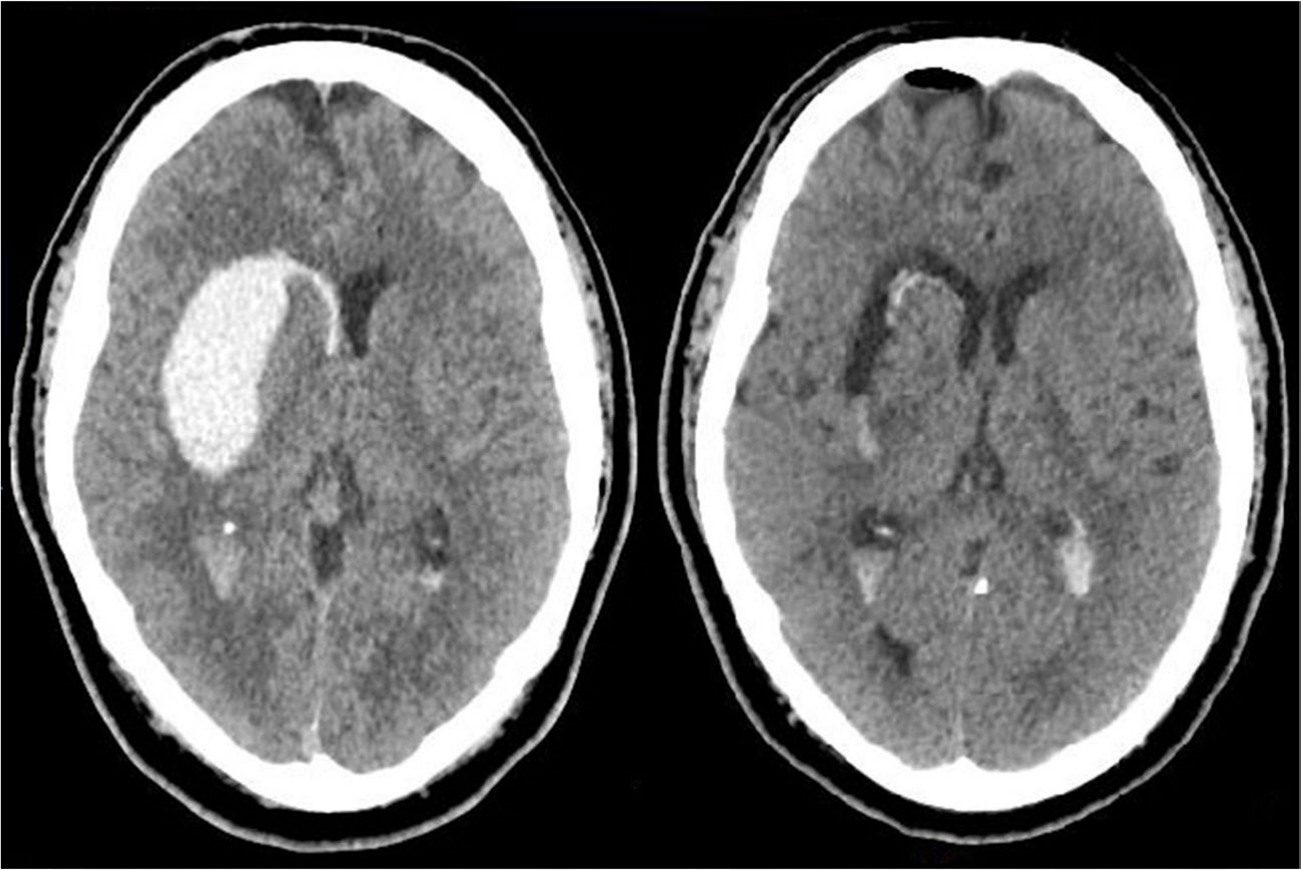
A 56-year-old man with a history of hypertension presented to the emergency room with a GCS score of E3M6V3, an NIHSS score of 21 and ICH-GS score of 9. NCCT showed a non-lobar haemorrhage on the right of 42.9 mL, with ventricular extension. CTA showed no spot sign. Surgery was started 7 h and 40 min after symptom onset. Postoperatively, the remaining volume was 3.0 mL, a reduction of 93.1%
